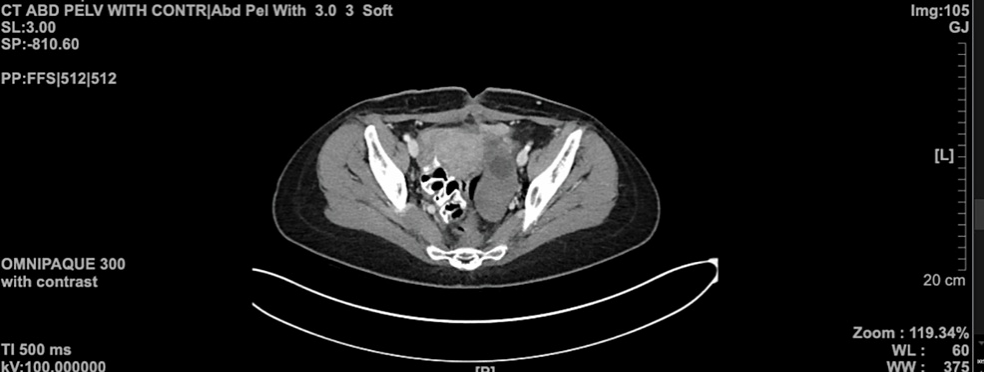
CT scan of the abdomen and pelvis conveying the resolution of the abscess CT: computed tomography
